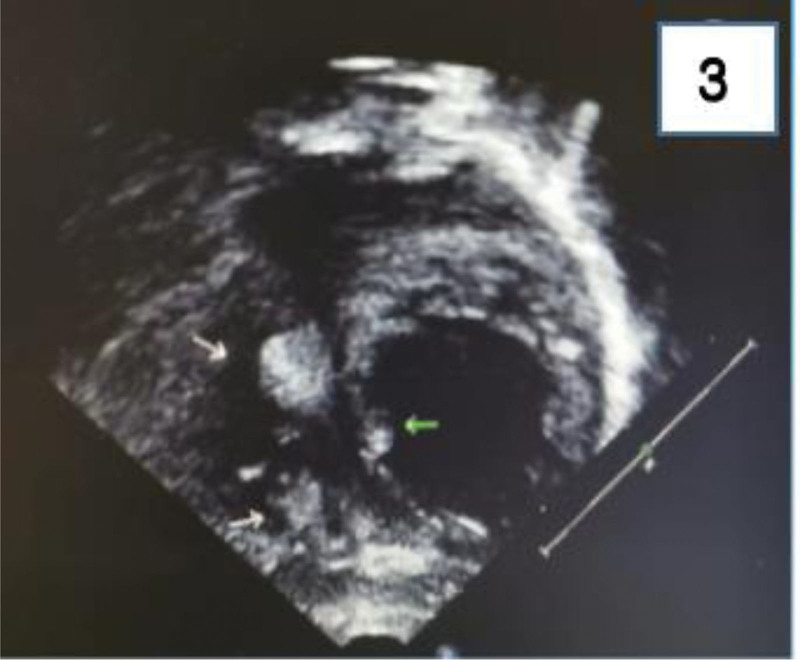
Echo enhancement of left and right ventricles and left ventricular outflow tract (multiple rhabdomyomas are possible) is shown by color Doppler echocardiography.
